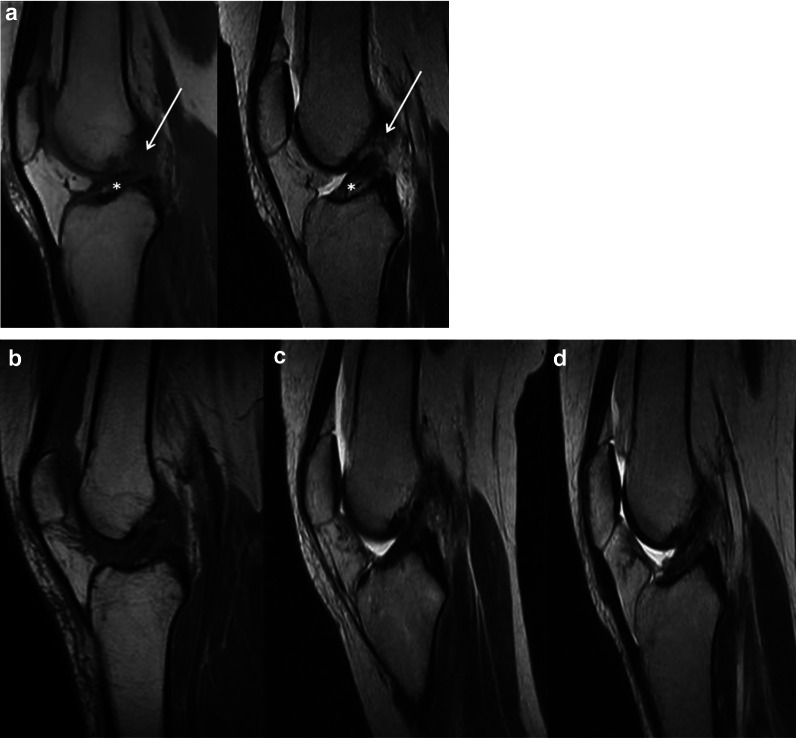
Pre- and postoperative images of patient #6. a Sagittal preoperative MRI T1-TSE (left) and T2-TSE (right). Tear location (arrow): type II; tissue quality (asterisk): good b Sagittal T2-TSE 1 month post-op. Morphology: grade 1 (normal); signal intensity: grade 1 (isointense) c Sagittal T2-TSE 3 months post-op. Morphology: grade 1 (normal); signal intensity: grade 1 (isointense) d Sagittal T2-TSE 6 months post-op. Morphology: grade 1 (normal); signal intensity: grade 1 (isointense) *TSE* turbo spin-echo
